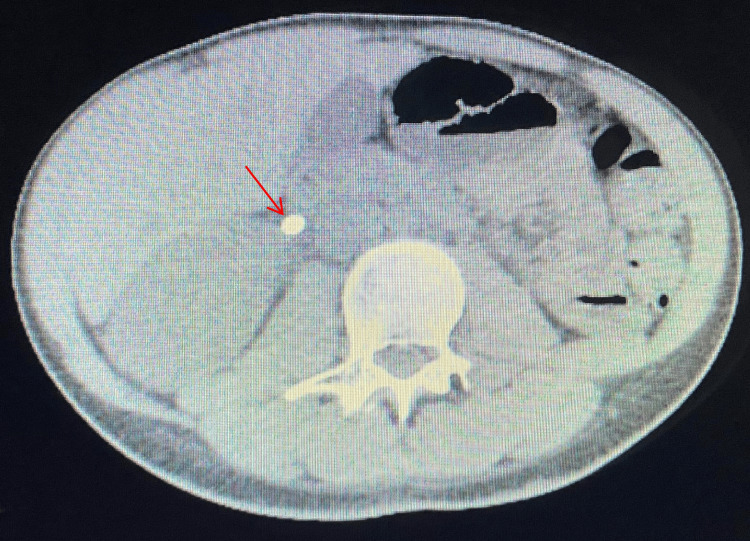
CT showing urinary stone in the right proximal ureter
